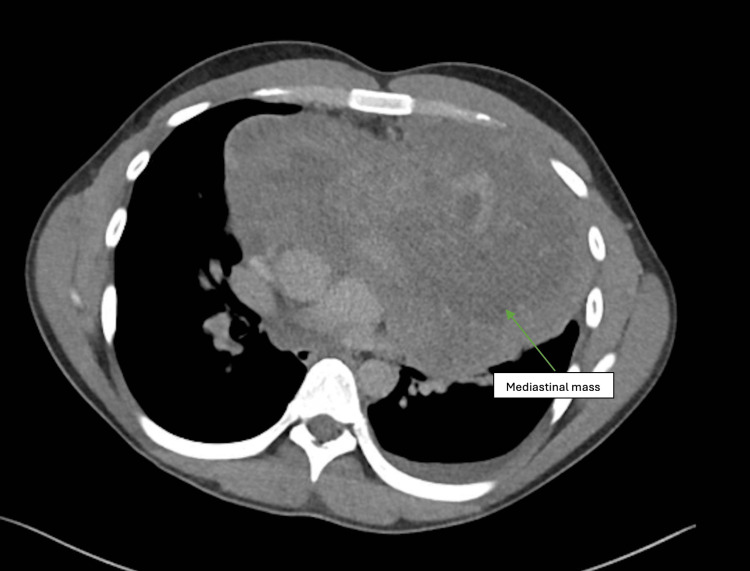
Axial contrast-enhanced CT thorax showing the anterior mediastinal mass bordering the pericardium, with associated left pleural effusion The appearances raised concern for local involvement and contributed to staging and treatment planning. The arrow highlights the anterior mediastinal mass, adjacent to the pericardium.
